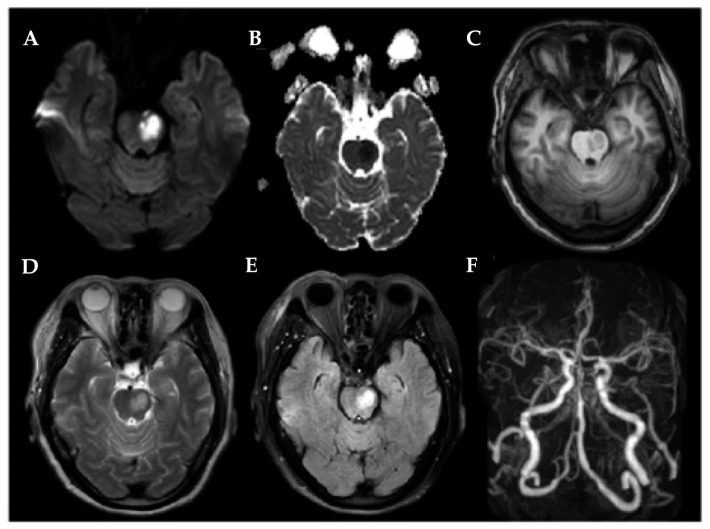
A 59-year-old female patient was referred for a left pontine infarction case. (A) High signal on DWI axial images; (B) low signal on ADC; (C) low signal on T1-weighted section; (D) high signal on T2-weighted section; (E) high signal on FLAIR; (F) the MRA 3D-TOF confirmed basilar artery stenosis.
